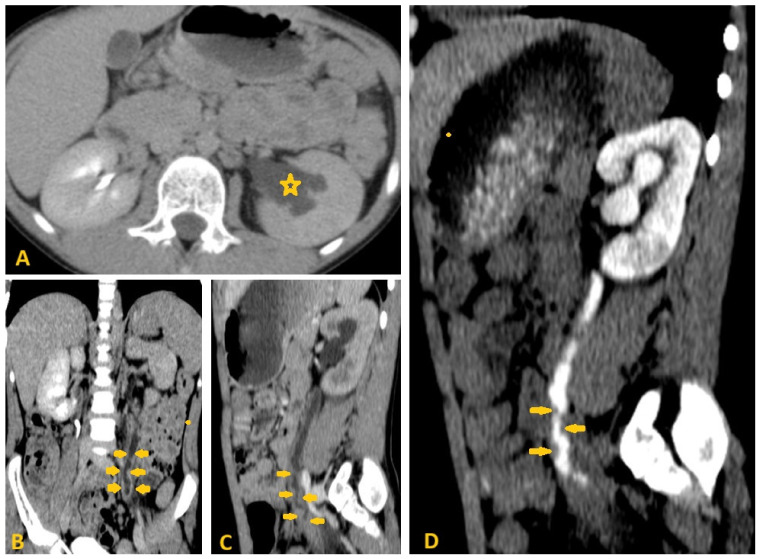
Selected post-contrast computed tomography images of a 10-year-old boy presenting with left abdominal pain show delayed excretion of contrast in the left kidney with moderate left hydroureteronephrosis (star on the axial image (A)) due to circumferential wall thickening at the middle part of the left ureter causing severe ureteric liminal narrowing (arrows on coronal reconstruction image (B) and sagittal reconstruction image (C)). Delayed sagittal reconstruction image (D) shows multiple filling defects in the narrowed ureter (arrows). This case was diagnosed as ureteric Schistosomiasis, and follow-up images showed improvement after *Schistosoma* treatment.
